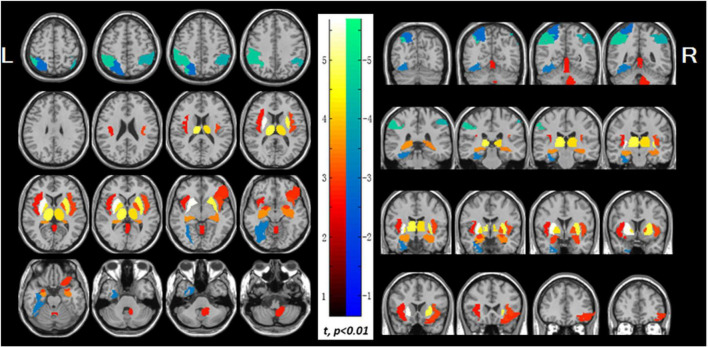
Abnormal glucose uptake in the non-small cell lung cancer patients. The increased regions display with red-to-white color, which include both left and right sides of the insula, putamen, pallidum, thalamus, hippocampus and amygdala, the right side of cerebellum, orbital part of right inferior frontal gyrus and vermis, while the decreased regions display with blue-to-green color, which include left superior parietal lobule, bilateral inferior parietal lobule, and left fusiform gyrus (*p* < 0.01). PET findings were overlaid on magnetic resonance image secondary. Color bar indicates *t*-values; L, left; R. right (Zhang W. et al., 2016).
